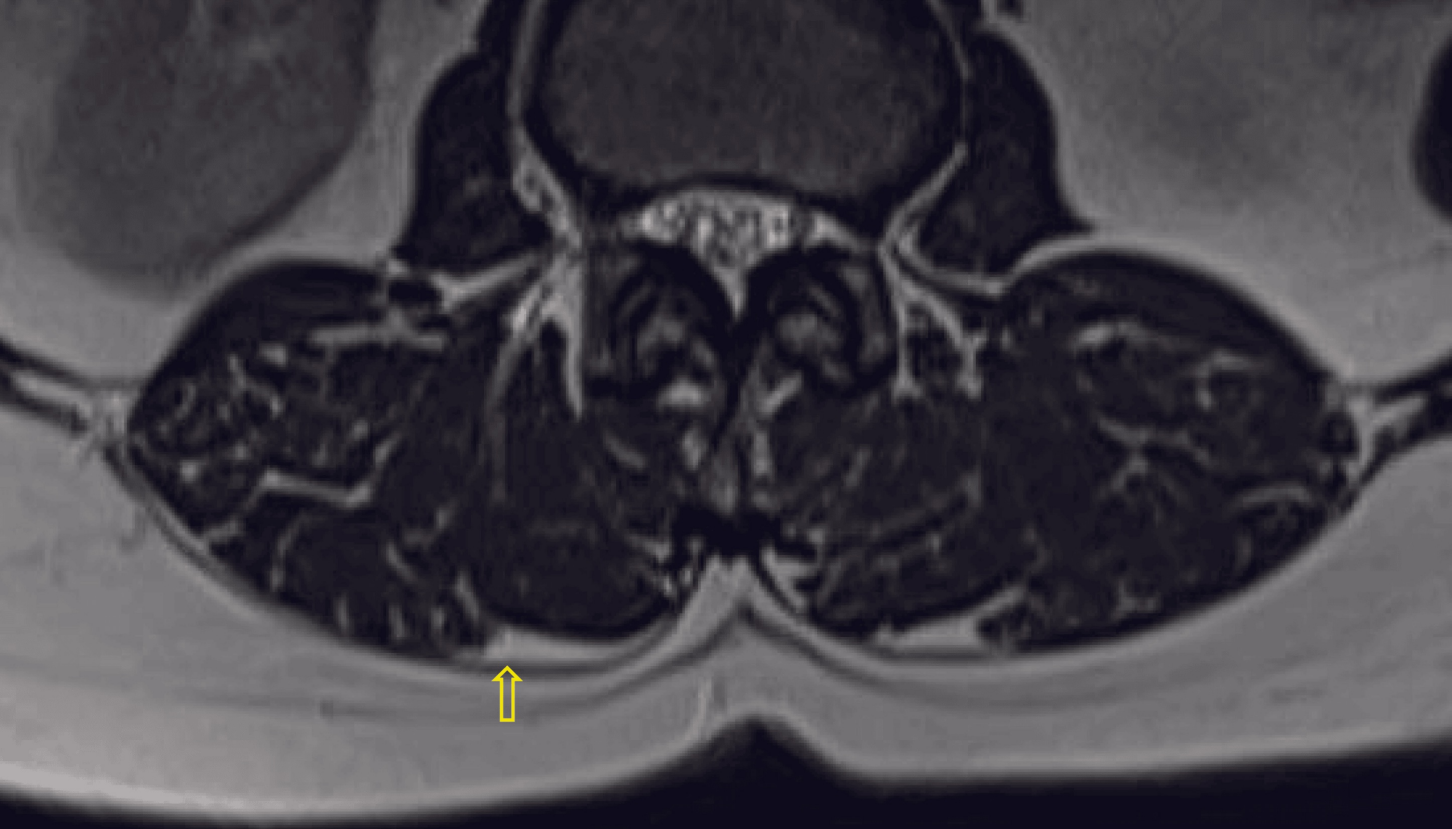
Axial T2-weighted MR image with yellow arrow pointing the epimuscular fat deposition along the posterior border of paraspinal muscles, with a score of 1 MR: magnetic resonance
